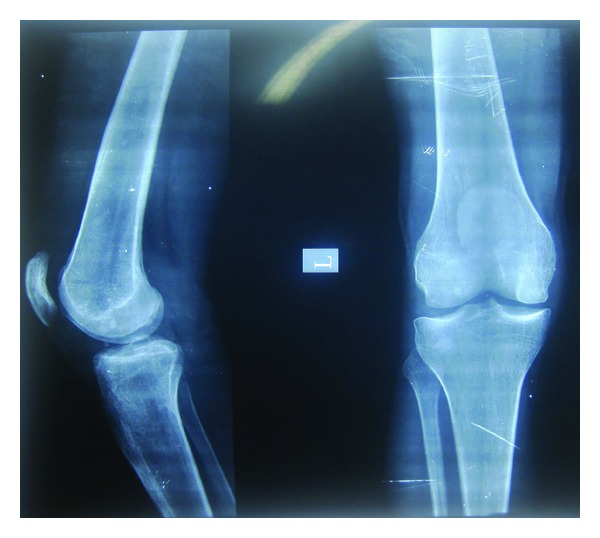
X-ray knee joint showing maldeveloped patella with osteoporosis in right side inferior pubic ramus, lower end of femur, and upper end of tibia.
